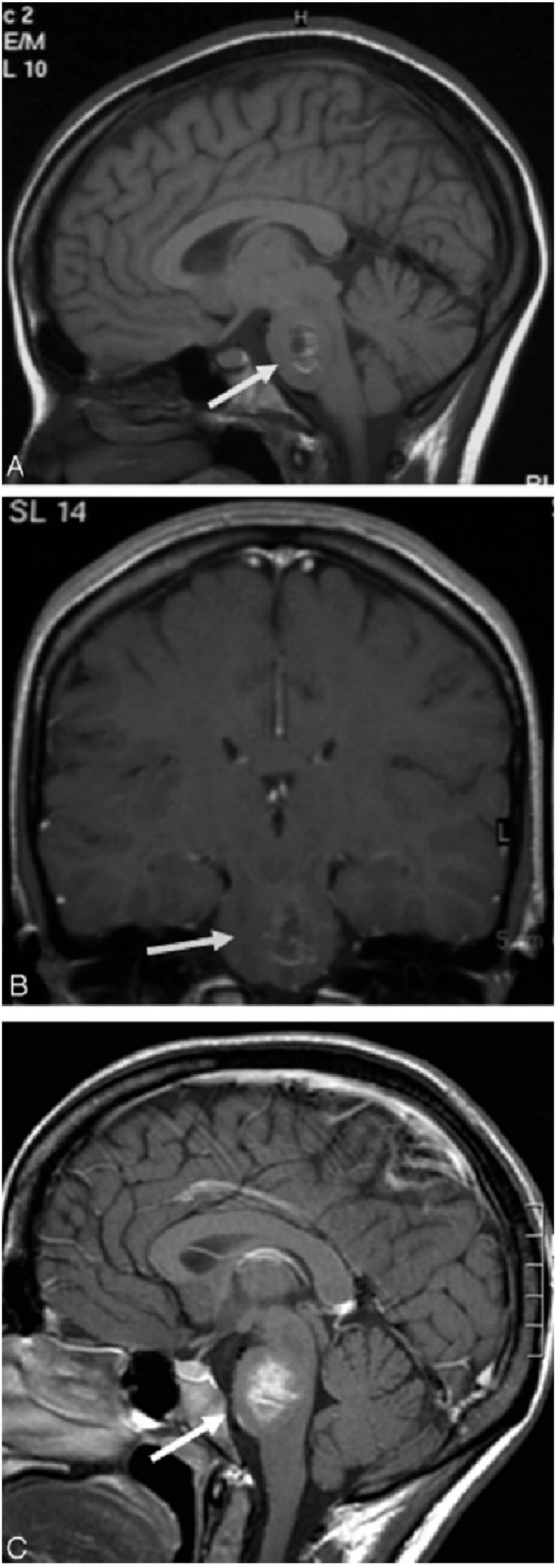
Patient with cavernous venous vascular malformation. Sagittal T1-weighted images without gadolinium (A) and coronal T1-weighted images with gadolinium (B) show no significant enhancement in the left pons lesion. However, 4 days later, T1 MR images (C) reveal prominent enhancement of the same lesion with ferumoxtran-10. Reproduction with the permission of reference (Manninger et al., 2005). Copyright ^©^2005 American Society of Neuroradiology.
